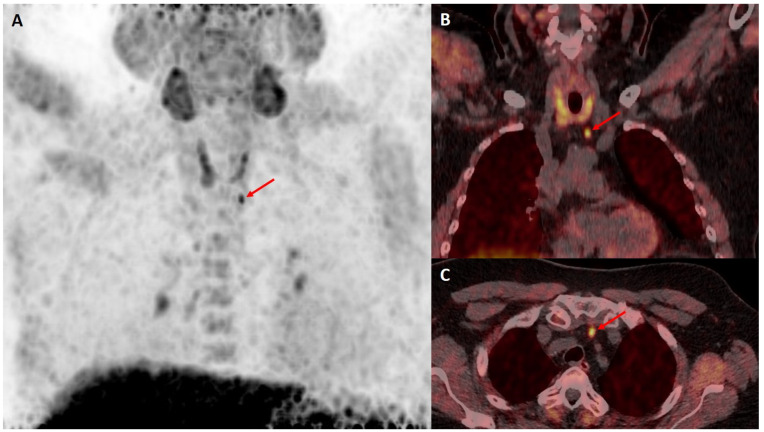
Imaging of a parathyroid adenoma, indicated by a red arrow, in a 65 year old female patient with normal calcium levels using [^18^F]fluorocholine PET/CT, showing (A): maximum-intensity-projection PET, (B): coronal PET-CT and (C): transaxial PET-CT slices.
